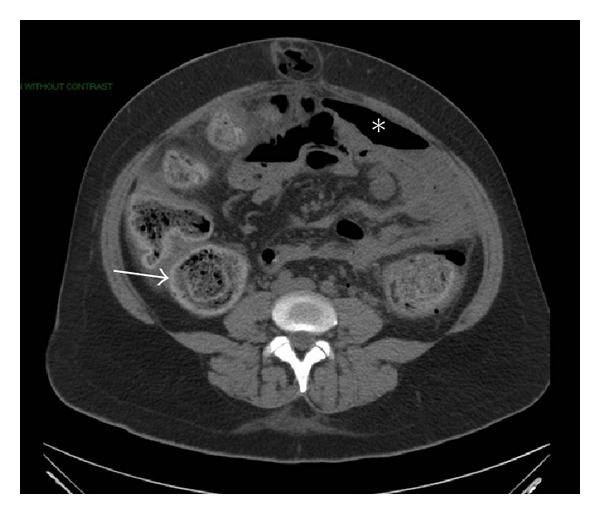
CT scan of the abdomen with free air (star) and colonic wall thickening (arrow).
